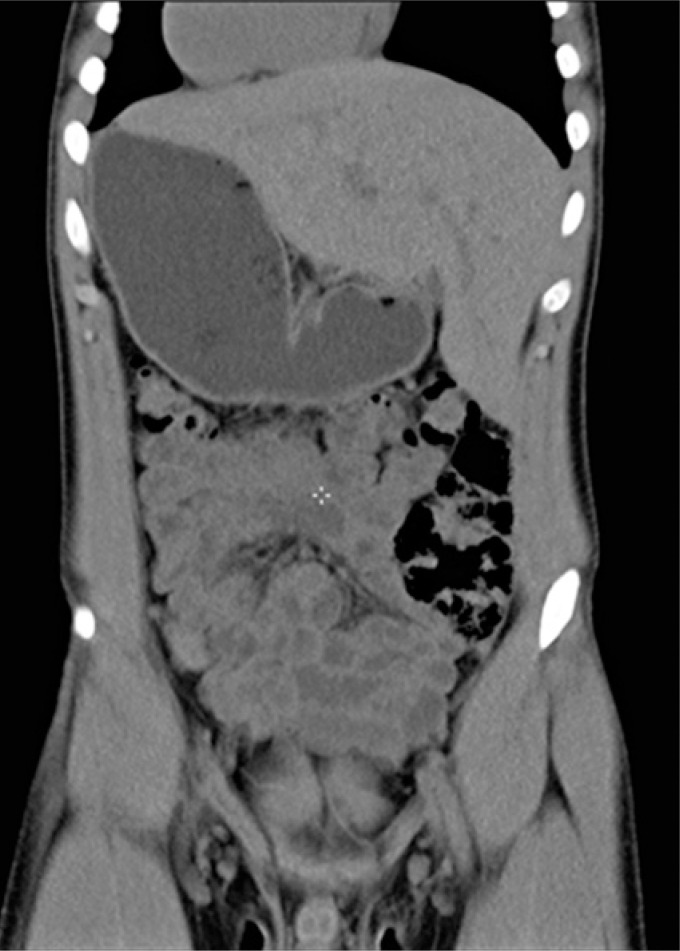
Computed tomography of the total abdomen in coronal view showing abdominal organs in a mirrored position relative to their normal topography
